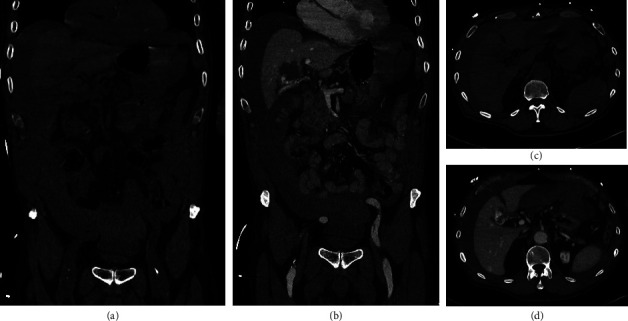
Computed tomography at admission. Computed tomography at admission is displayed. Frontal images of native (a) and venous (b) phase, as well as transverse images of native (c) and venous (d) phase, are shown.
